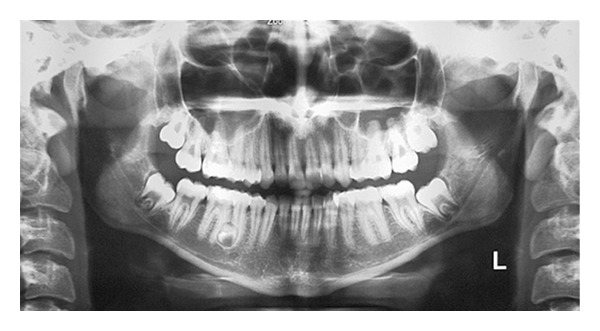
Panoramic radiograph taken at 19 years of age showing development of a supernumerary premolar.
